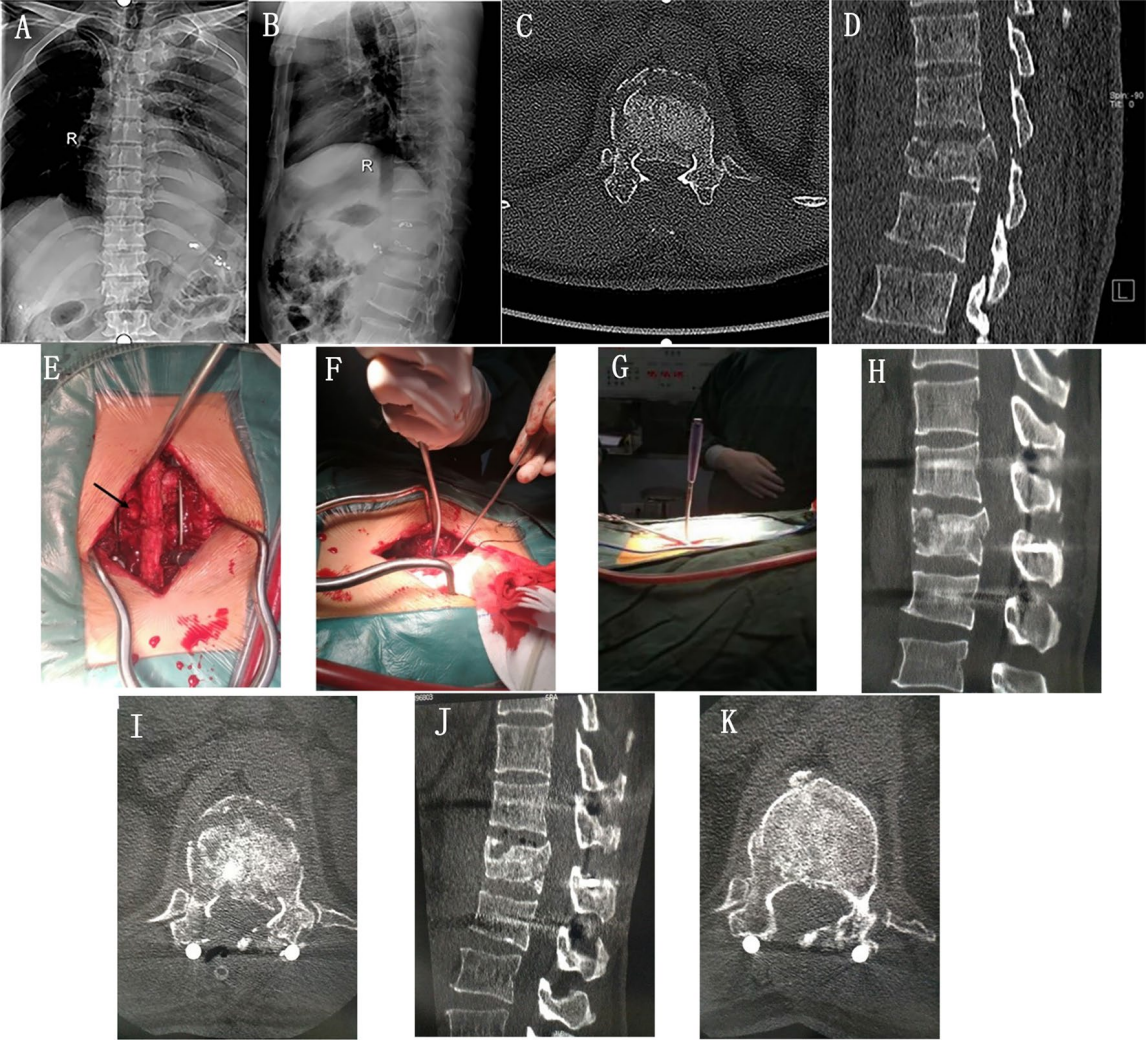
A 46-year-old male patient with a T_12_ burst fracture and spinal cord swelling injury (ASIA grade D). A and B are the anterior and lateral X-rays of the spine before surgery; C and D are preoperative CT coronal and sagittal images; E, F, and G refer to the use of Y-shaped fracture mass reduction device during surgery, with the arrow in E showing the size of the lamina space fenestration during surgery; H and I are the coronal and sagittal CT images immediately after surgery; J and K are the coronal and sagittal CT images from the last follow-up
